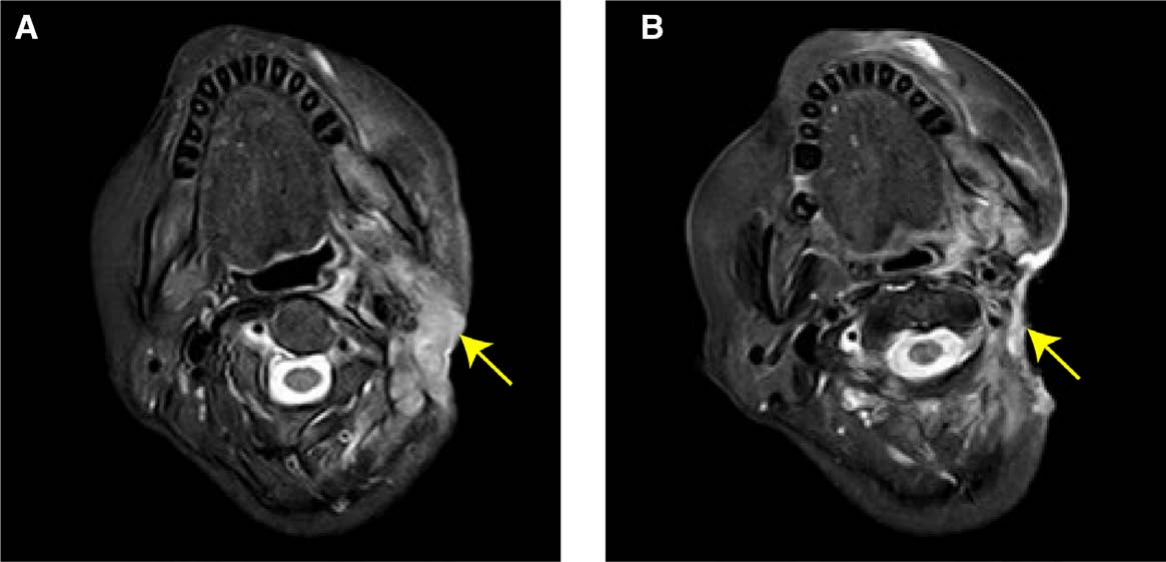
MRI scan of case 1 before and after use of pm-Pac combined therapy. The lesion was in the postoperative area around left middle ear, with the inner edge reaching the left side of the nasopharynx and the outer edge reaching the left outer auricle. It shows uneven enhancement in T1 enhanced signal. (A) The lesion progressed into 5.8 cm × 2.9 cm, time: 2023-01-07; (B) after 6 cycles of pm-Pac combined therapy, the lesion reduced to 3.3 cm × 1.0 cm, time: 2023-07-27. MRI = magnetic resonance imaging.
